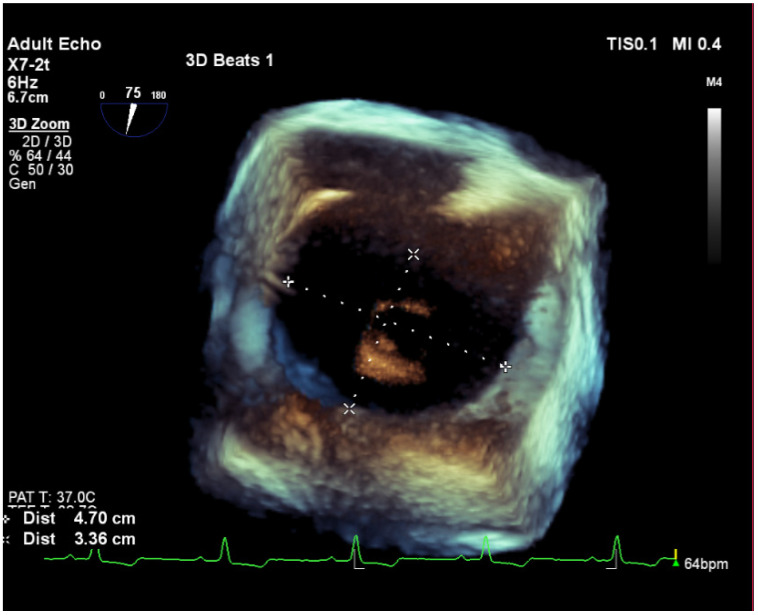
3D Transesophageal echocardiography showing the size of the atrial septal defect.
